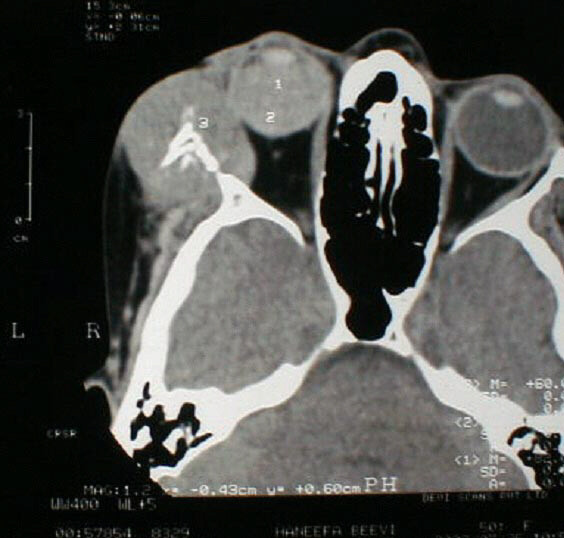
Computed tomographic scan showing two separate lesions one in right orbit and other in right zygoma, zygomatic process of maxilla.
